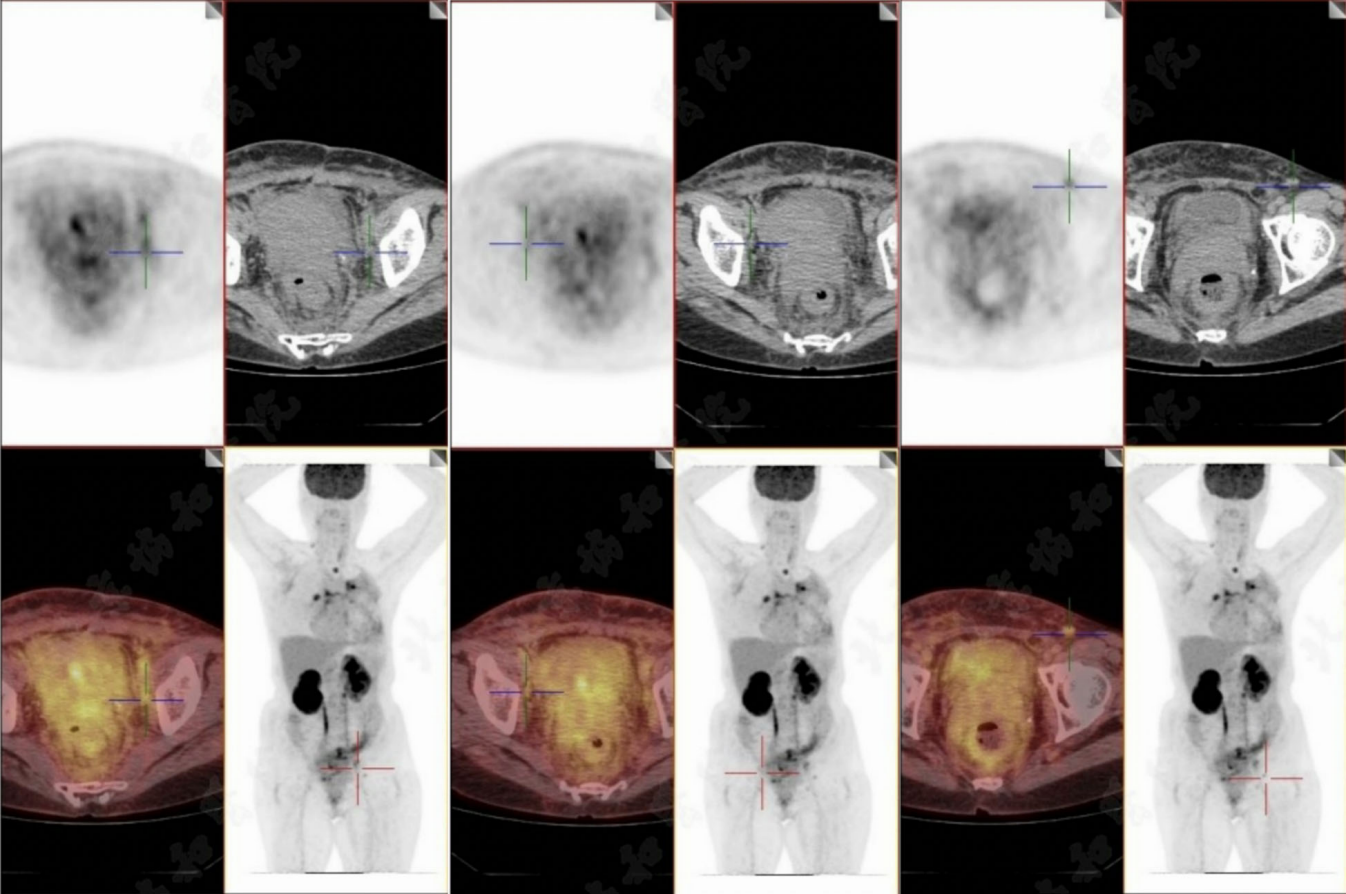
PET-CT imaging of abdominal area. PET-CT scan conducted on September 26, 2024, reveals multiple lymph nodes with increased radioactive uptake located around the abdominal aorta, near the bilateral iliac vessels, and in both inguinal regions. The largest lymph node, measuring approximately 1.1 cm in short diameter, demonstrates a maximum standardized uptake value (SUVmax) of 4.3.
